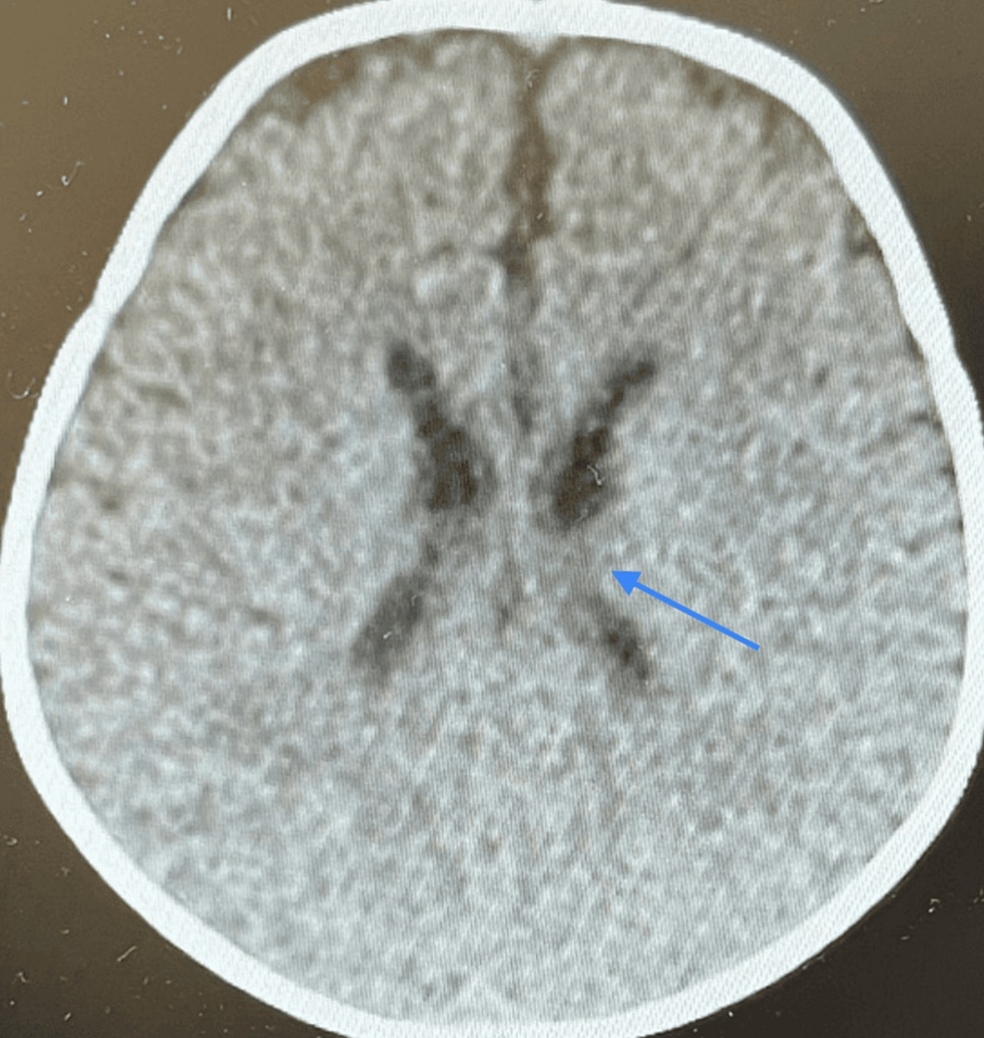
Non-contrast CT brain scan: thin corpus callosum.
